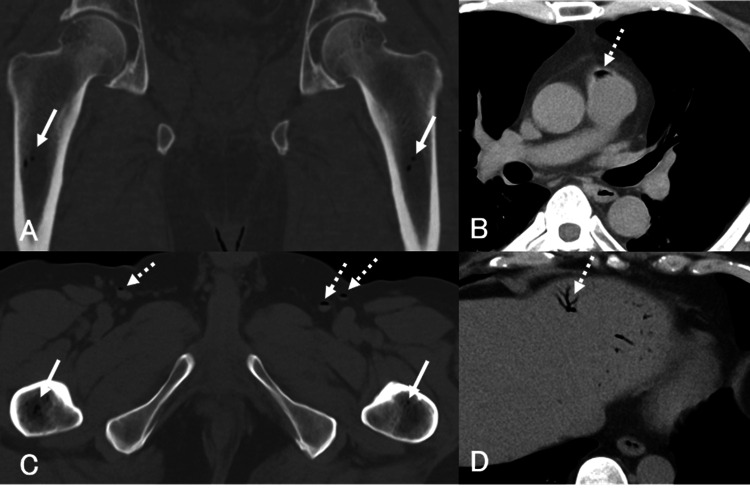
Case 2 Pre-hyperbaric oxygen therapy (HBOT) plain computed tomography (CT). A, C: Bone window settings (A: coronal view; C: axial view). All CT images were reviewed using a bone window setting with a window width of 3000 HU and a window level of 400 HU. B, D: Mediastinal window settings. Intramedullary gas is identified bilaterally in the proximal femoral bone marrow (solid arrows). Multiple gas densities are also observed in the pulmonary artery (B), femoral vein (C), and portal vein (D) (dashed arrows).
